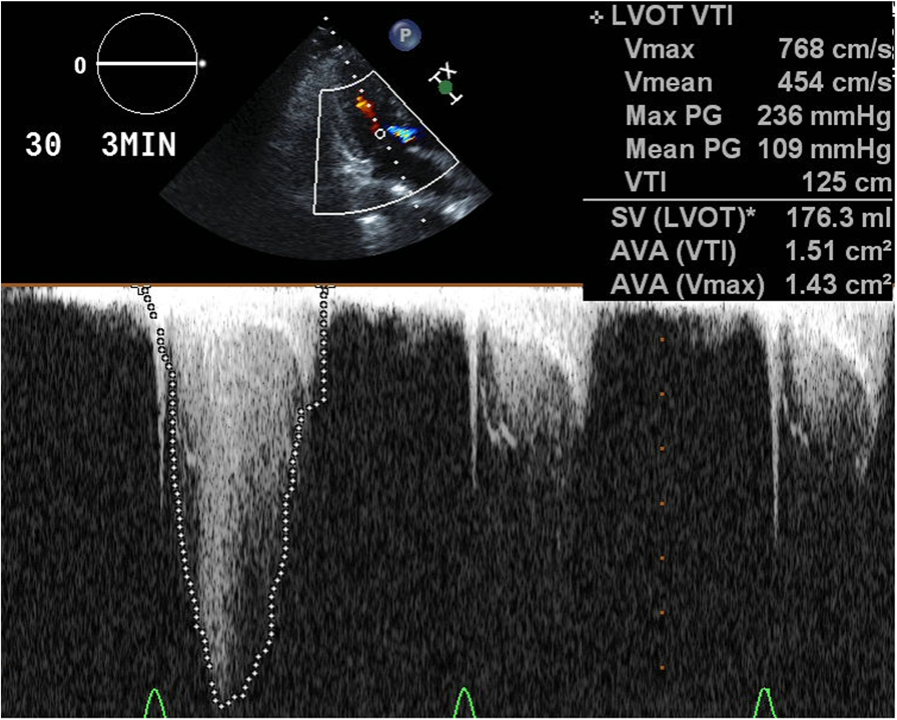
Provocation with dobutamine infusion (30 μg/kg per minute) during Doppler echocardiography on day 26. Echocardiography reveals a left ventricular outflow gradient of 236 mmHg, which confirms a diagnosis of latent left ventricular outflow tract obstruction. *LVOT* left ventricular outflow tract
